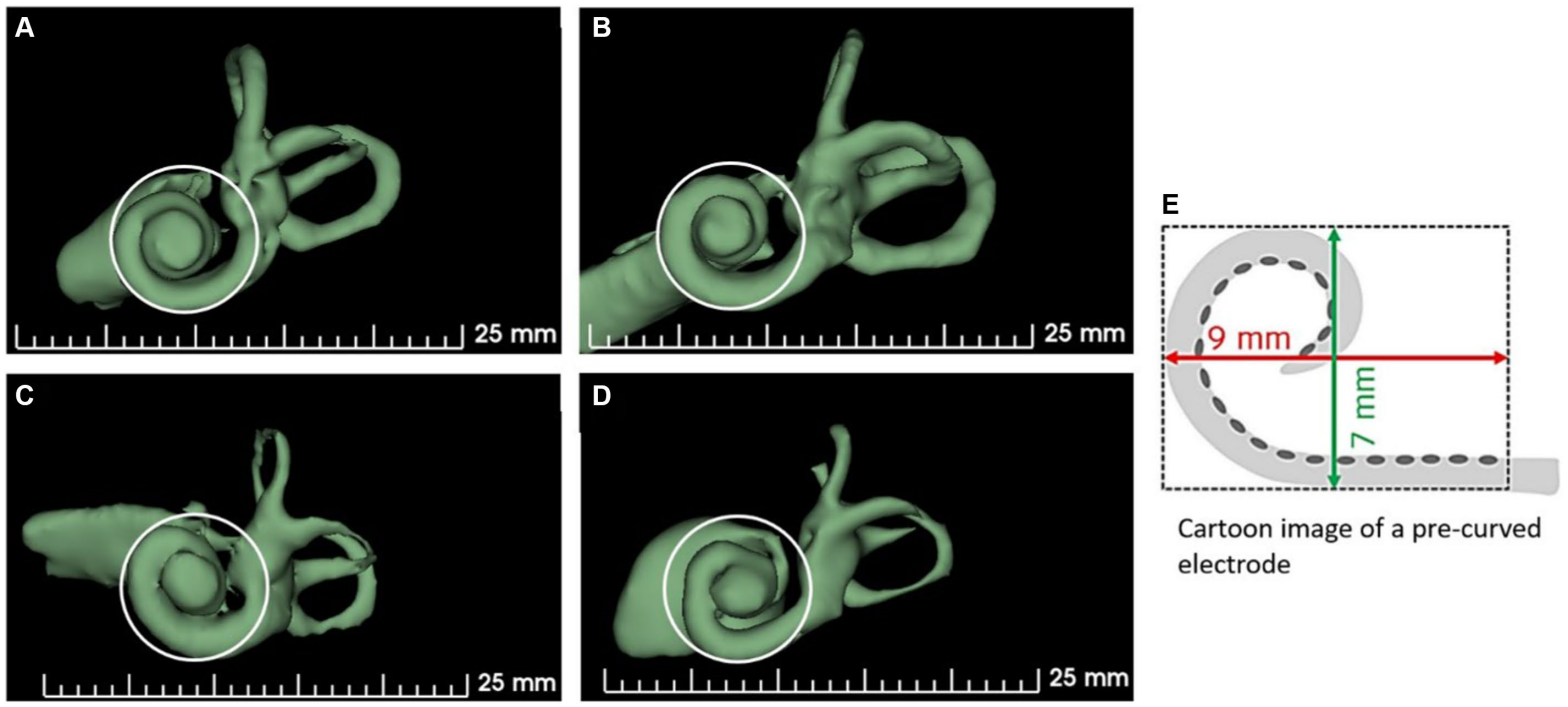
Shape variations in the cochlear basal turn. (A) More circular, (B) elliptical, (C) extended elliptical-shaped, (D) triangular, and (E) a perimodiolar electrode with a fixed size and shape. 3D segmented inner ear from CT scans of cochleae with varying shapes of basal turn are from anonymized subjects from St. Petersburg ENT and Speech Research Institute, Russia (33).
